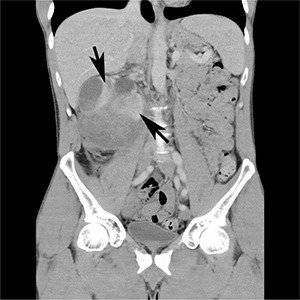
CT scan – coronal view showing right kidney mass with subcapsular haematoma (arrows).
